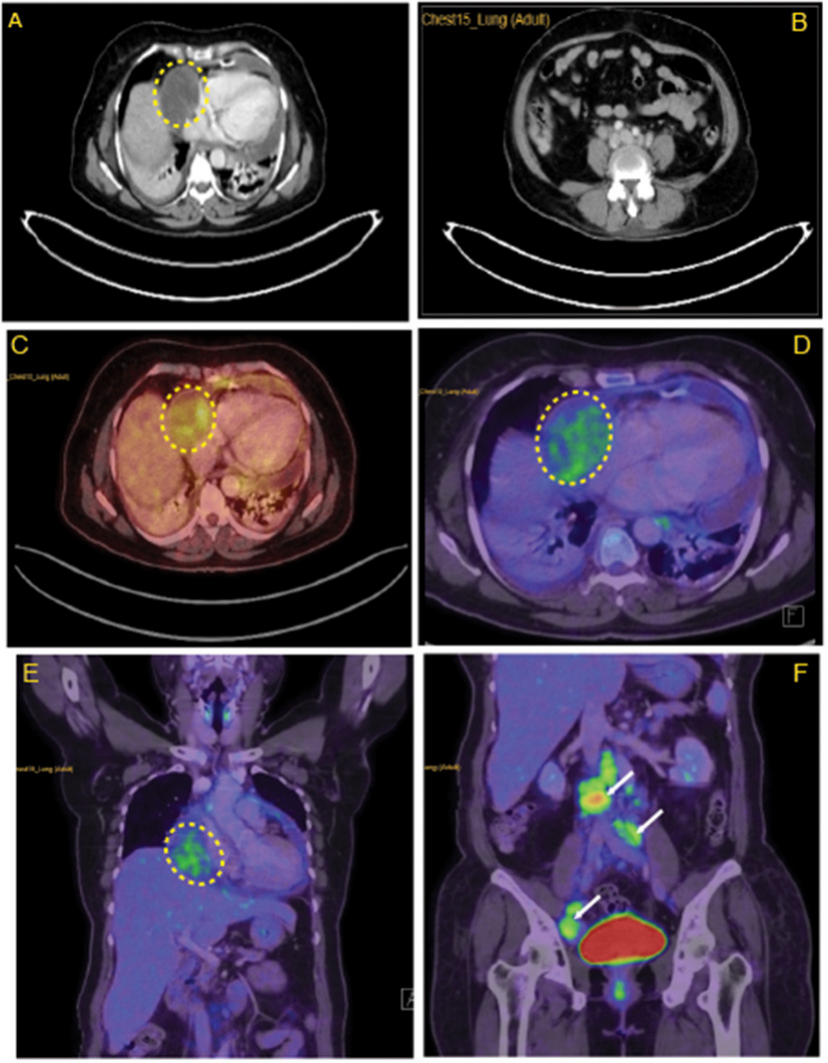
Lymph node characteristics in the right atrium. (A) CECT thorax revealing minimally enhancing mass lesion in the right atrial wall. (B) Multiple enlarged retroperitoneal lymph nodes. (C) Horizontal sections of FDG PET scan revealing mildly FDG avid hypodensity (5.8 × 5 cm, SUV max −3.40) along the lateral wall of the right atrium with mass effect over it. (D) Coronal section of FDG PET scan revealing mildly FDG avid mass over the lateral wall of the right atrium. (E) Enlarged FDG avid lymph nodes at perivascular, aortocaval, retrocaval, and bilateral common iliac and external iliac levels (F). Yellow highlighted area locates the myxoid liposarcoma in the right atrium. White arrows in (F) indicates metastasis in abdomen areas.
